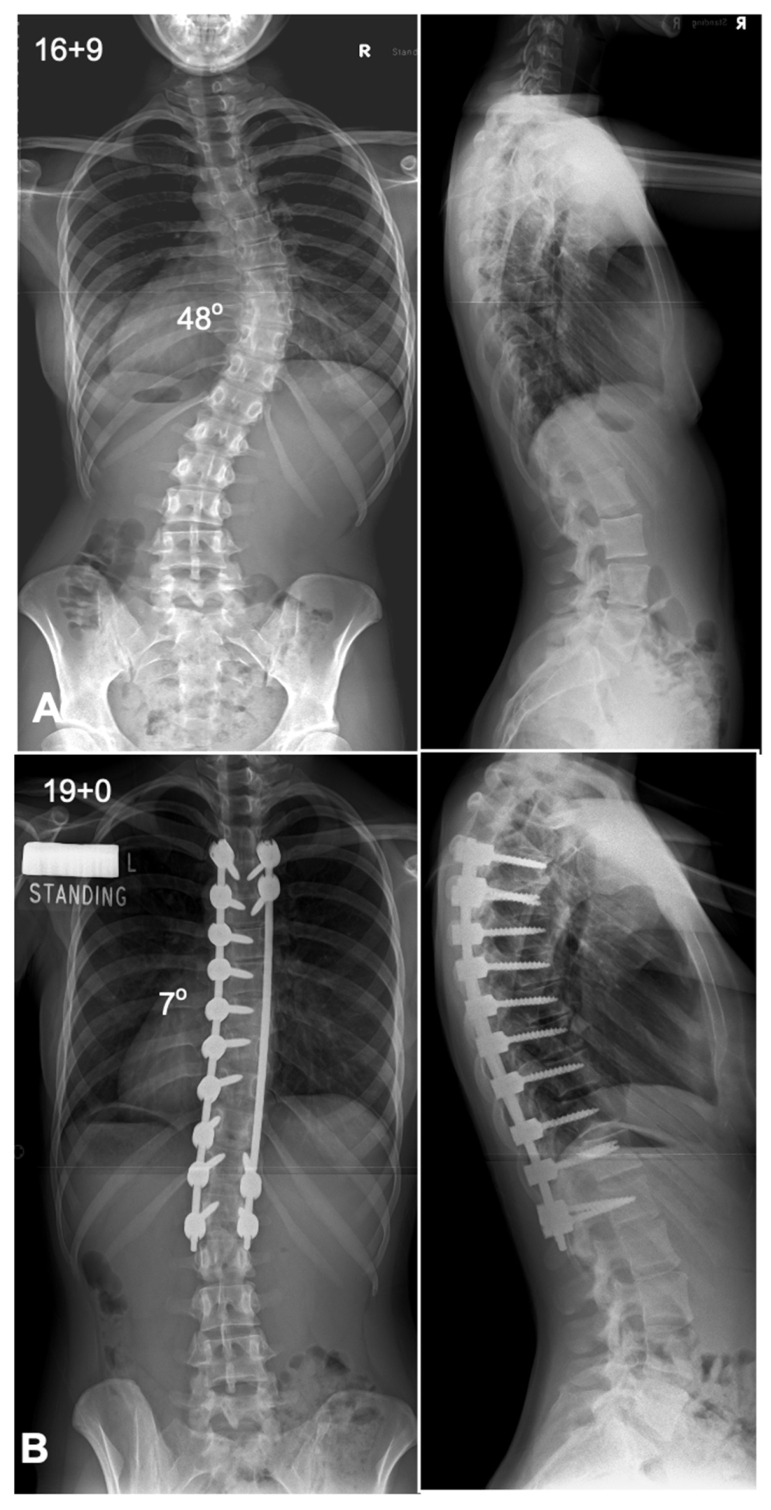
Deformity correction using a typical concave side rod derotation maneuver. (A) Preoperative antero-posterior and lateral radiographs showing a thoracic scoliosis with a Cobb angle of 48 degrees. (B) Postoperative radiographs showing good deformity correction with coronal and sagittal balance.
